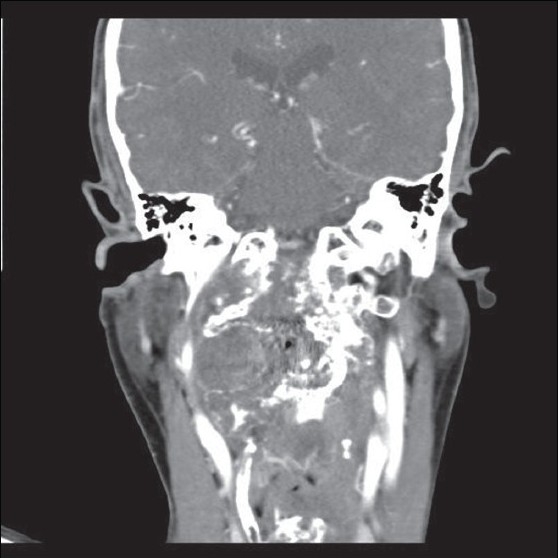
CT Scan (coronal view) showing the lesion
